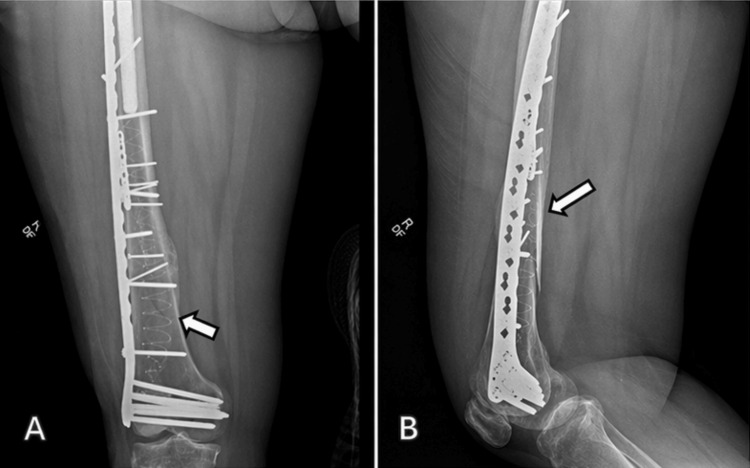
Six-month follow-up radiograph demonstrating a well-fixed and well-positioned construct with continued fracture healing No evidence of hardware failure or loosening.
